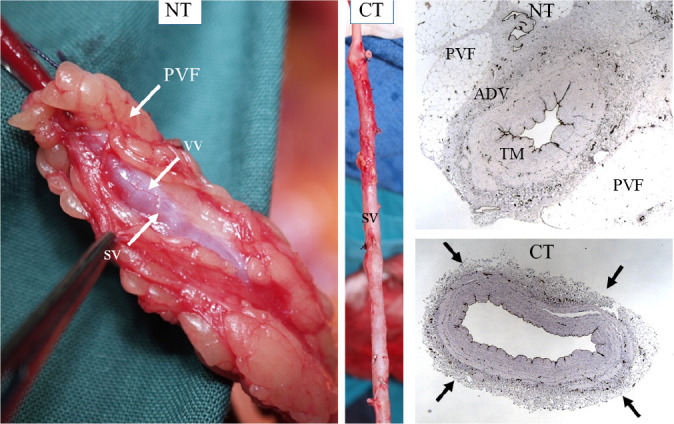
Vascular damage; no-touch (NT) versus conventional (CT) saphenous
